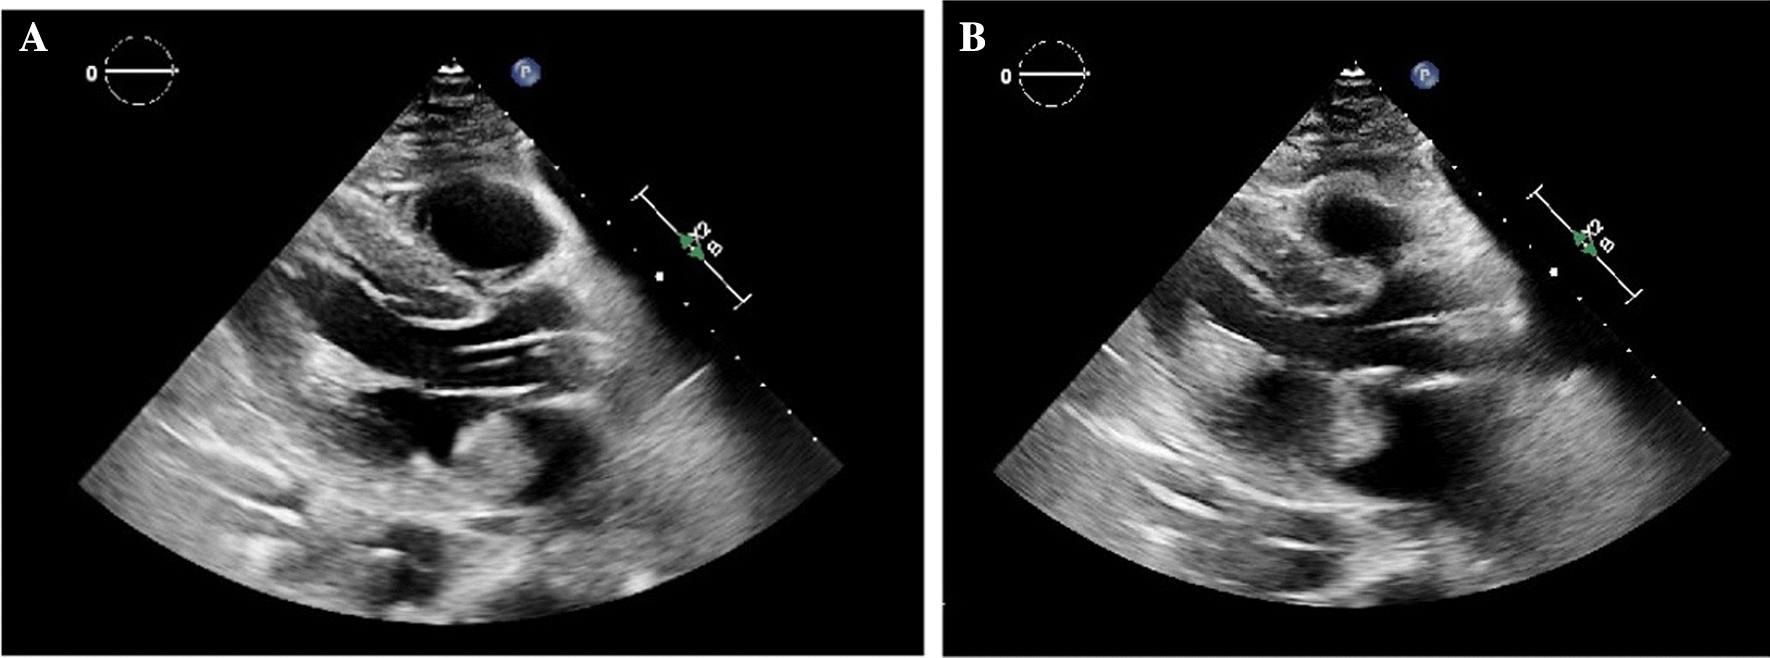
A, B: Transthoracic echocardiogram in the parasternal long axis view demonstrating a large left atrial mass intermittently obstructing the mitral valve; A (left image) demonstrating the left atrial mass in diastole, not obstructing the mitral valve; B (right image) showing the left atrial mass obstructing the mitral valve in systole
